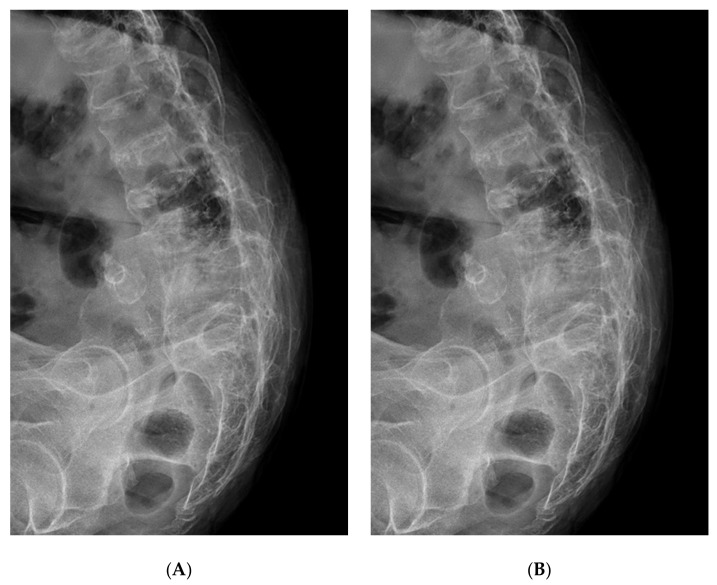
Flexion (A)-extension (B) lateral plain radiograph prior to cord injury.
